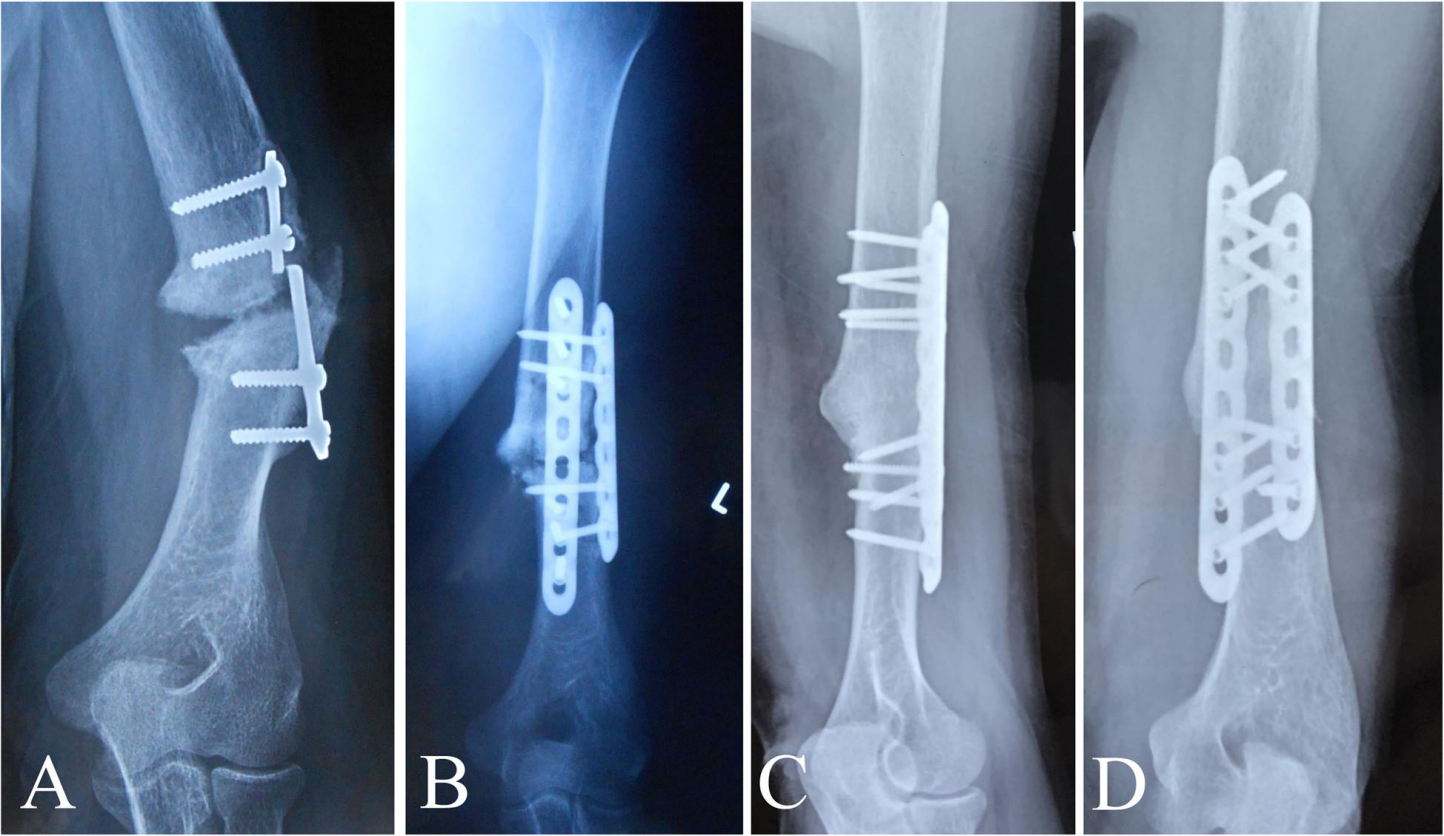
Images from a 58-year-old man who underwent plate fixation of a left humerus shaft fracture 30 years ago and was treated with plate fixation combined with autogenous iliac crest bone grafting because of nonunion 1 year postoperatively. However, the humeral fracture remained un-united until he visited our institution. a Preoperative plain radiograph showing classic synovial pseudarthrosis nonunion of the left humerus with a broken plate. b Radiograph taken immediately after revision surgery showing double locking compression plating with autogenous iliac crest bone grafting. c, d Radiographs showing consolidated bone union at 45 months after the index surgery
